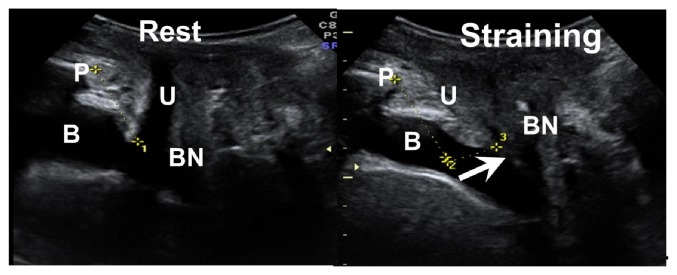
The sagittal view of perineal sonography. The pubic bone marked as P. The urethra marked as U. The bladder marked as B. The bladder neck marked as BN. The pubic bone is set as basic point and makes a line from the pubic bone to BN at rest and during straining. The maximal distance of two lines is calculated as bladder mobility.
